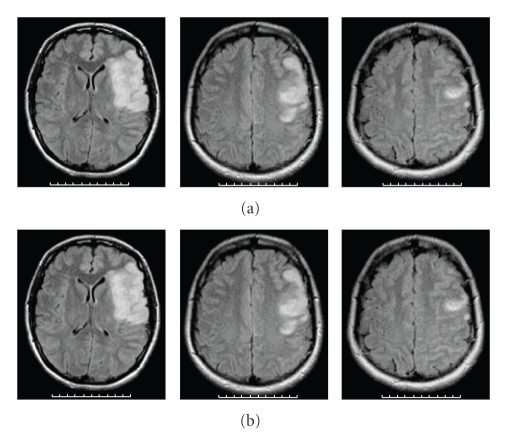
MRI T2 flair-weighted images showing left superficial MCA infarct. (a) 24 hours after stroke onset, (b) 5 days after stroke onset.
